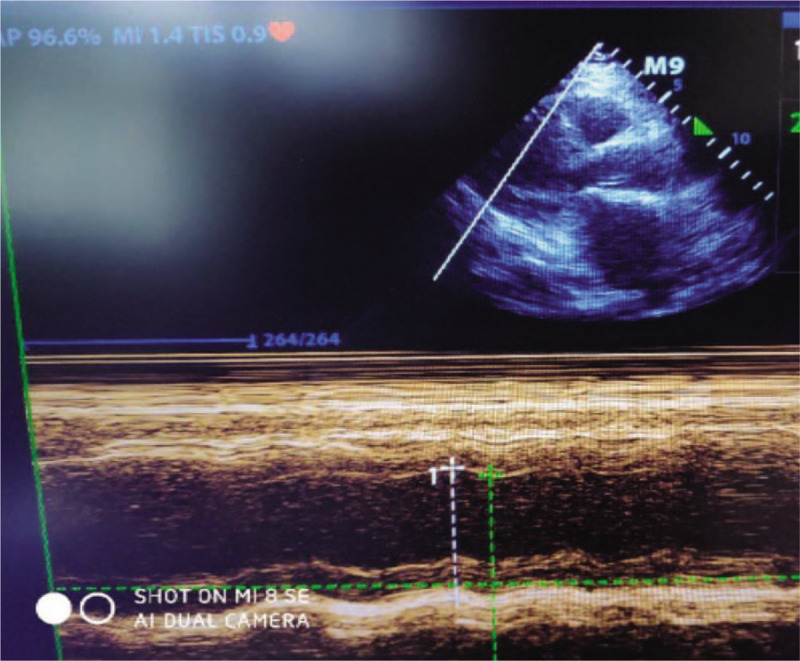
Echocardiogram demonstrated diffuse reduction of left ventricular wall motion, the left ventricular ejection fraction (EF%) was 23.5%.
